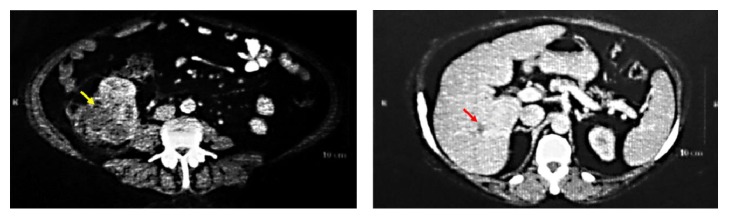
CT of Abdomen. Yellow arrow: mass attached to the right kidney (yellow arrow) on CT scan with bear paw sign. Red arrows: probably liver hemangiomas.
